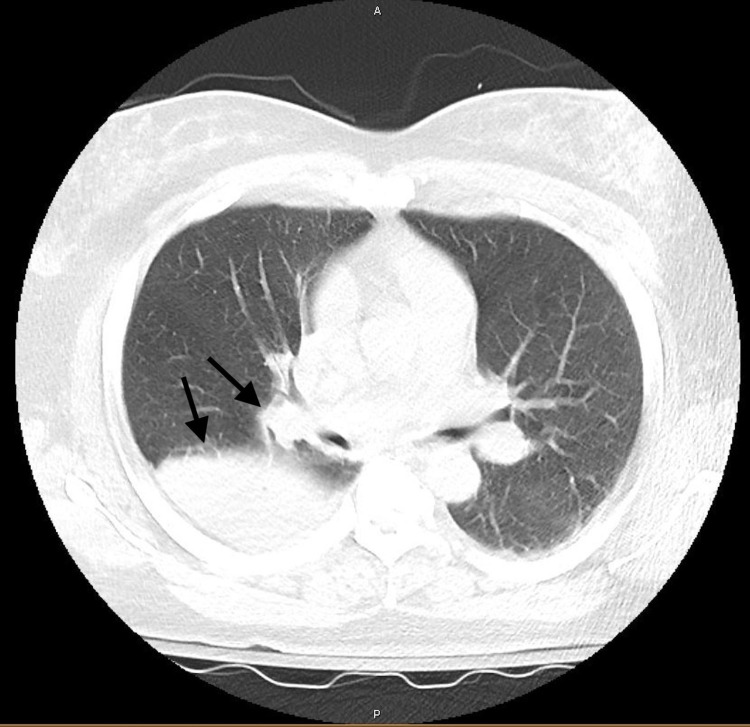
CTA Chest Right Upper Lobe CTA: Computed tomography angiography
